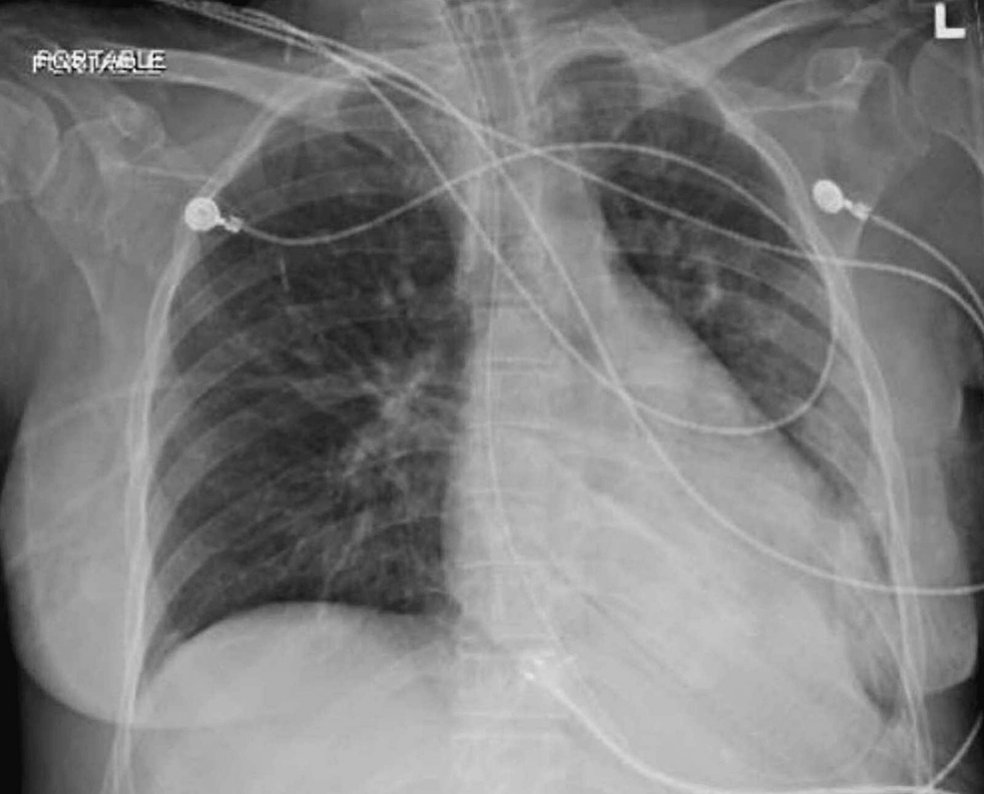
Chest X-ray after pericardiocentesis showing significant reduction in cardiac silhouette after drainage of pericardial fluid.
